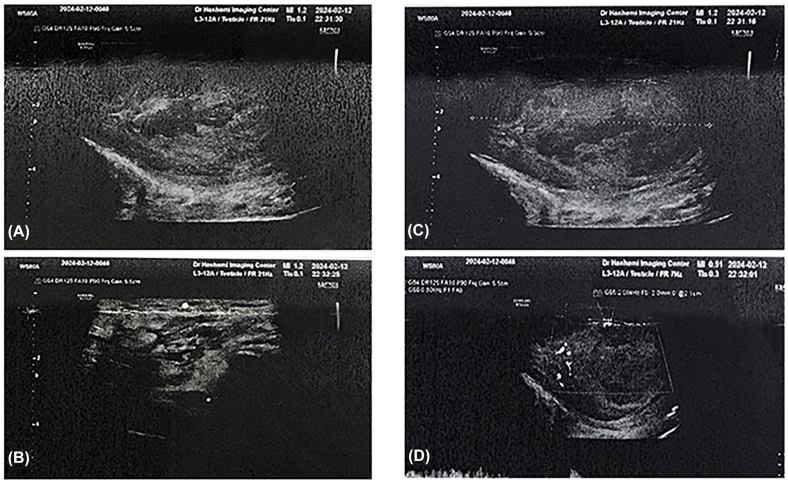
(A, C) Ultrasound of the enlarged right testis with hypoechoic areas suggesting abscess formation. (B) Thickened spermatic cord with soft tissue edema. (D) Peripheral vascularity indicating necrosis.
